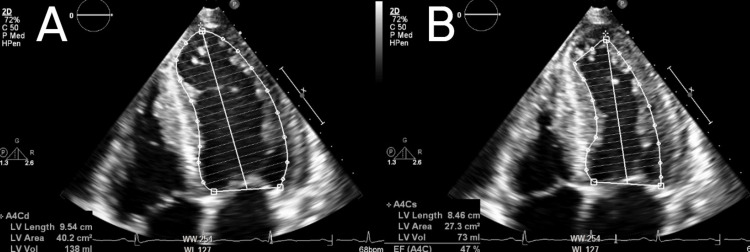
Apical four-chamber view which was used in Simpson's biplane method of disks to estimate mildly reduced left ventricular ejection fraction of 47% on echocardiogram. (A) Image shows left ventricular end-diastolic volume; (B) image shows left ventricular end-systolic volume.
